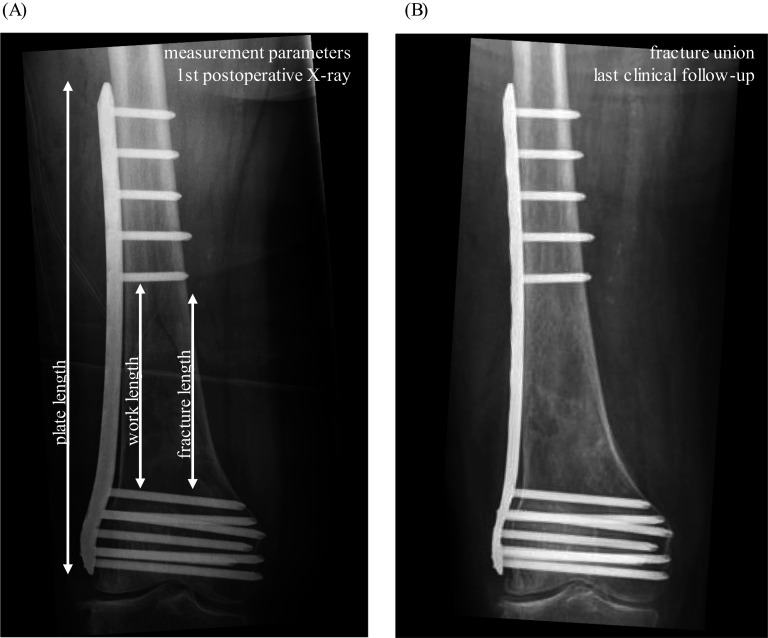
X-ray example: measured parameters and example of fracture union
